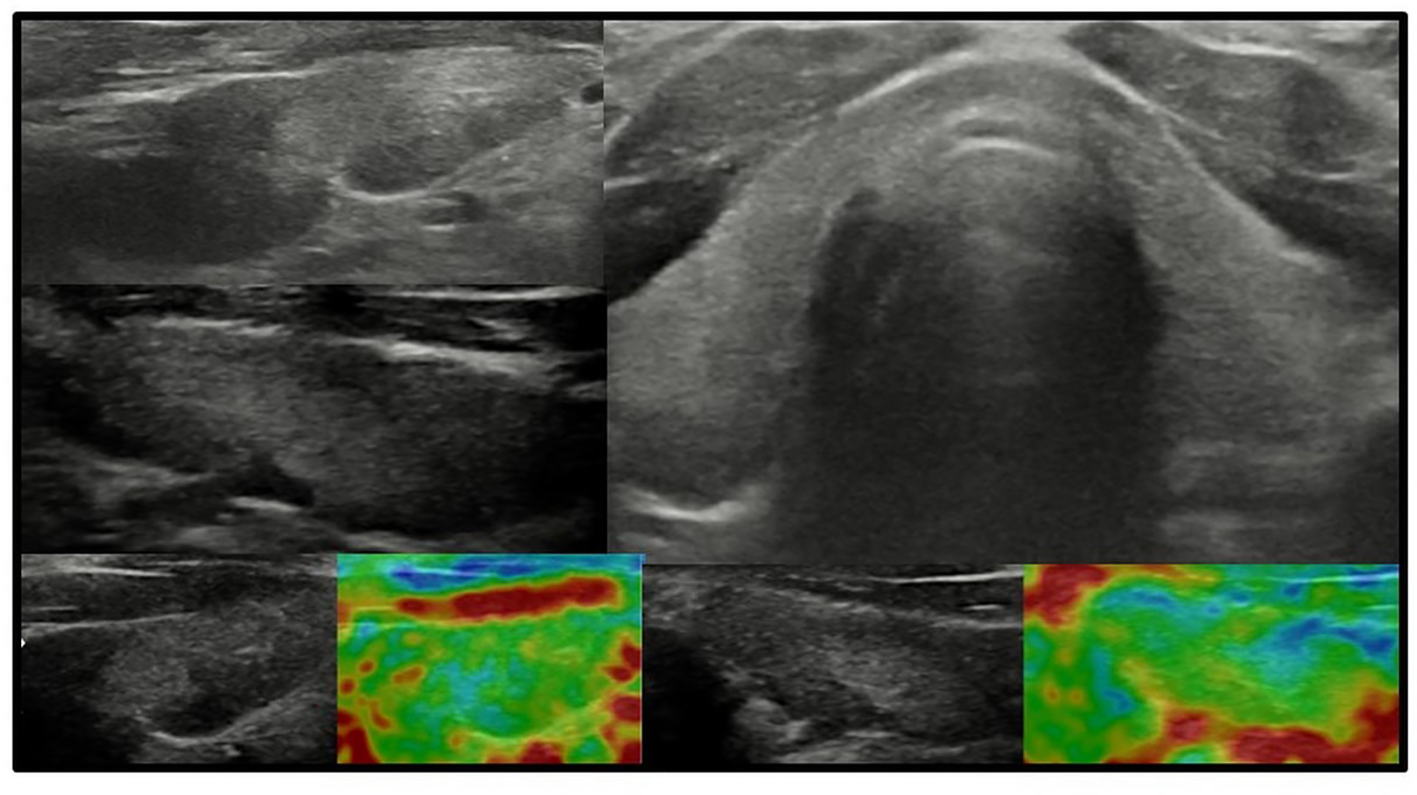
Axial and longitudinal ultrasonography scans of the thyroid gland in patient F2 presenting with irregular echostructure and echogenicity of the thyroid gland. Elastography reveals that the hyperechogenic areas are stiffer than the peripheral areas of the thyroid gland. Blood flow is normal. Samsung HS40.
